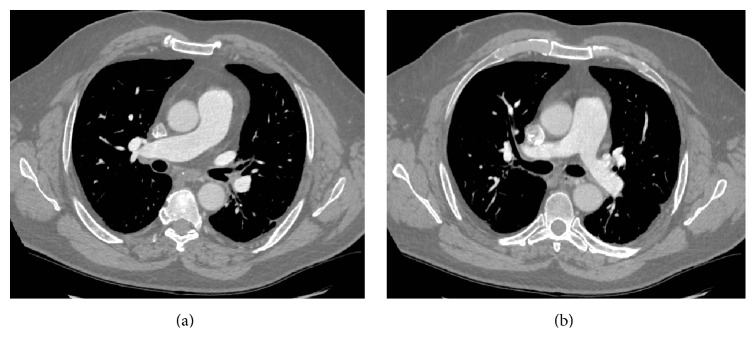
Contrast-enhanced CT pulmonary angiogram showing normal opacification of right and left pulmonary arteries. No evidence of pulmonary embolism.
